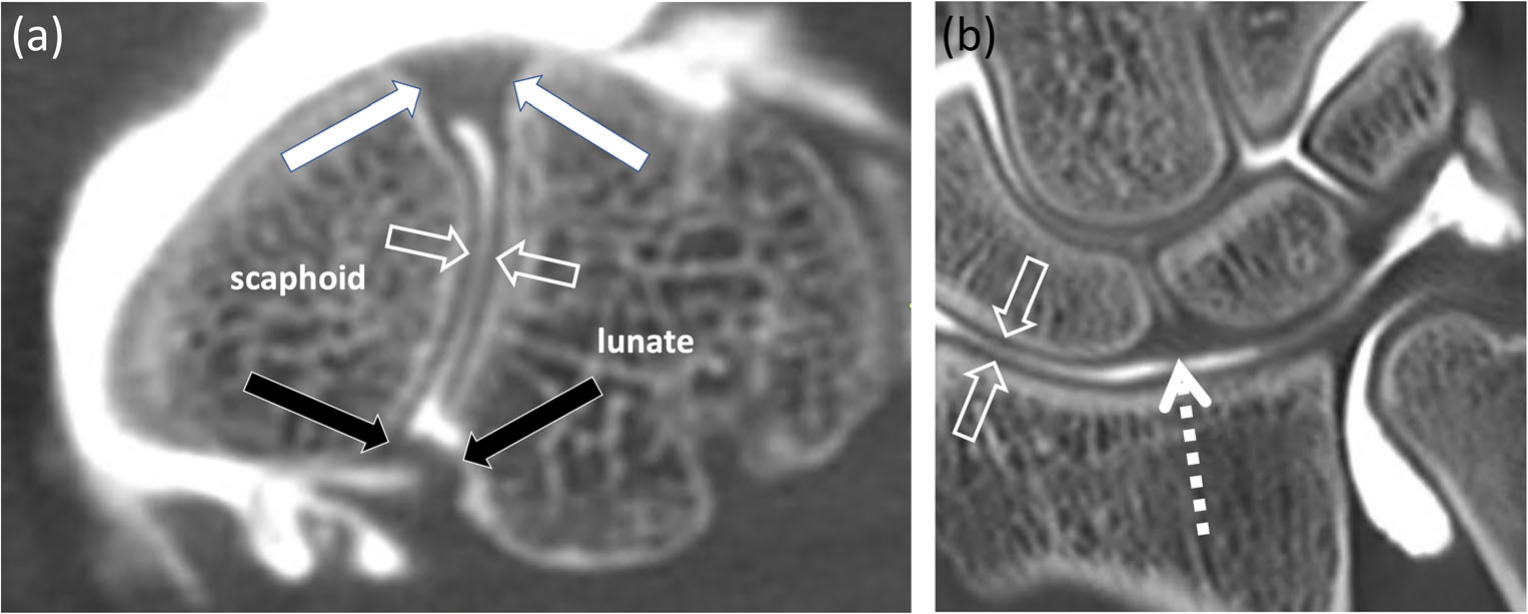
A 20-year-old male patient underwent tricompartmental CT arthrography to assess the scapholunate ligaments after a wrist trauma. CT arthrography demonstrates normal findings with continuity of the palmar band (a, black arrows), the dorsal band (a, white arrows) and proximal/membranous band (b, dashed arrow) of the scapholunate ligament on transverse (a) and coronal images (b). Open arrows (a, b) indicate regular articular cartilage in both imaging planes
